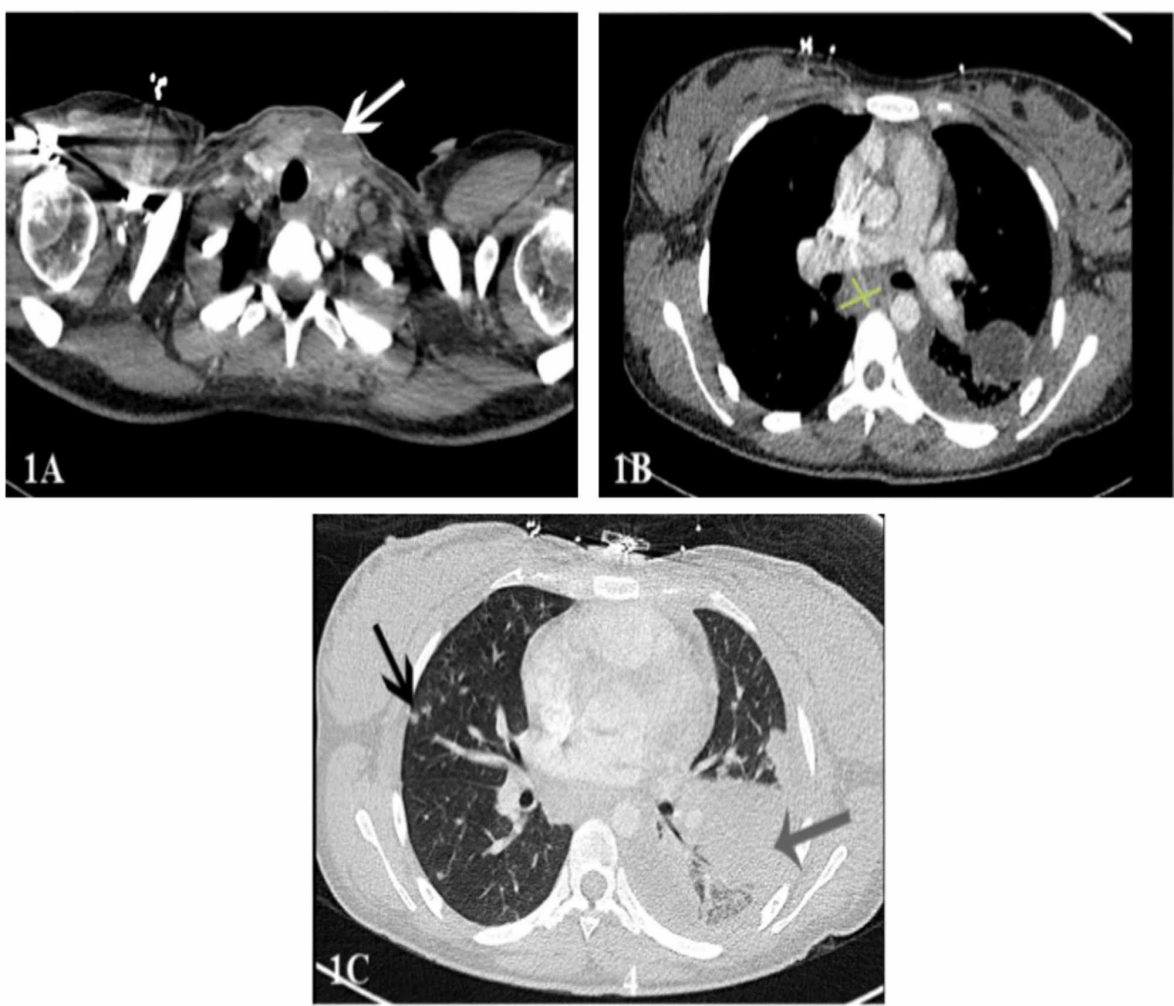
Initial CT chest with contrast (A) A 3.2 cm x 2.4 cm mass-like density within the left thyroid lobe. (B) Multiple mediastinal lymph nodes with the largest being subcarinal measuring approximately 1.7 cm x 3.6 cm. (C) Left lower lobe consolidation and a small pleural effusion (gray arrow). Additionally, throughout bilateral lungs, there are many subcentimeter solid noncalcified nodules (black arrow).
